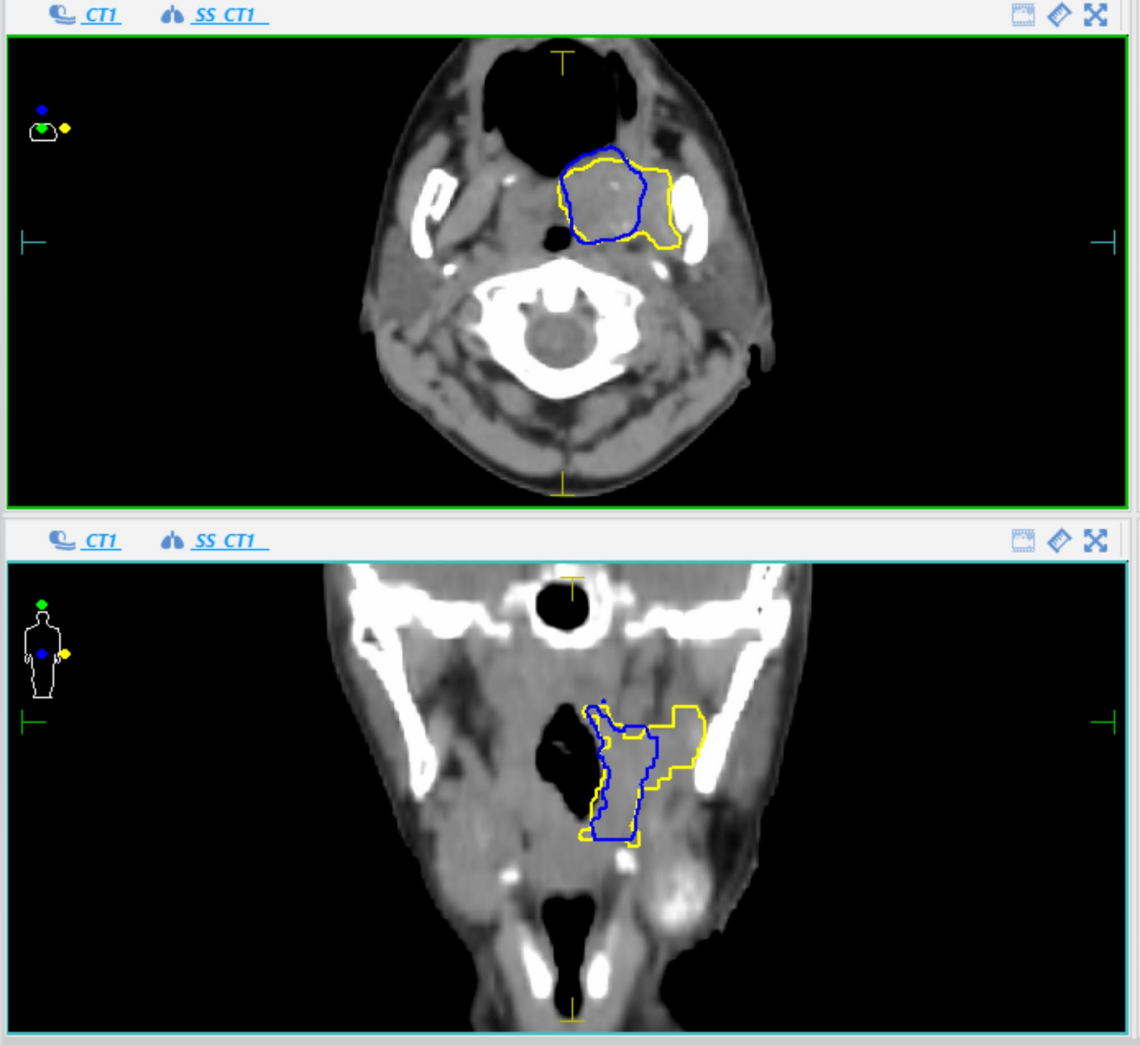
An example of “relevant change”. Blue = CT contour, Yellow = MRI contour
